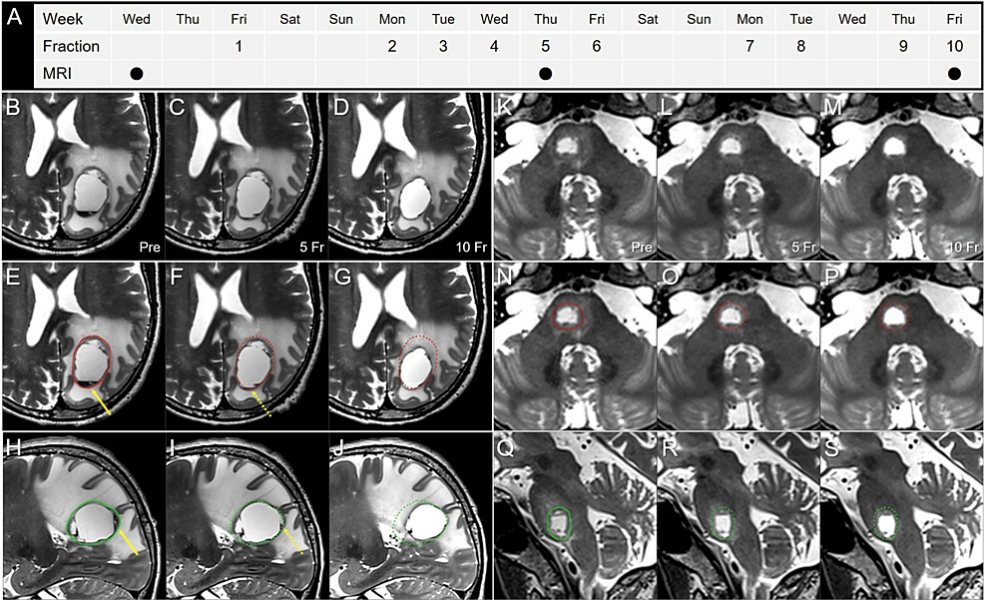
T2-weighted images obtained two days before stereotactic radiosurgery, at 5 and 10 fractions. The images show (A) the actual schedule for fractionated SRS (fSRS) and MRI acquisition; (B-J) the parietal lesion; (K-S) the pons lesion; (B-G, K-P) axial images; (H-J, Q-S) sagittal images; (B, E, H, K, N, Q) before fSRS (pre); (C, F, I, L, O, R) at 5 fraction (fr); and (D, G, J, M, P, S) at 10 fr. These T2-weighted image datasets were co-registered to the dedicated workstation, MIM Maestro^TM^ (Cleveland, OH: MIM Software) and then fused to coincide with each other through the cranium. The pre-fSRS GTVs were contoured in red and green for the axial and sagittal images, respectively. In the parietal lesion, slight dorsomedial displacement of the lesion along with disappearance of the preexisting fluid sedimentation (arrows in E, H) was observed at 5 fr (dashed arrows in F, I). At 10 fr, a 39% (7.71 cm^3^) reduction in the GTV (19.93 to 12.22 cm^3^) and dorsomedial shifting of the tumor center were noted along with an improvement in the mass effect. Slight evisceration of the GTV dorsomedially from the initial dimensions was found ad eundem at 5 and 10 fr. In the pons lesion, no significant change was observed, apart from regression of the mild surrounding edema.
